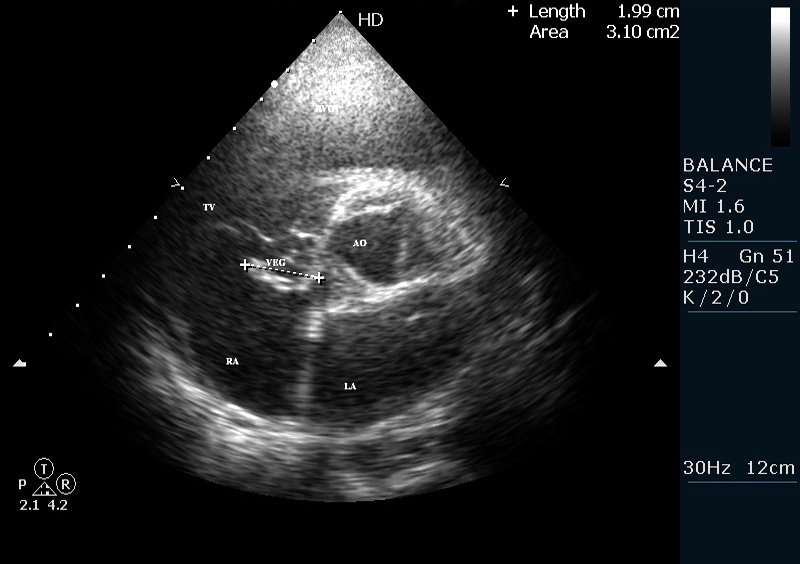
Transthoracic short axis parasternal view measuring the vegetation about 20 mm long.
